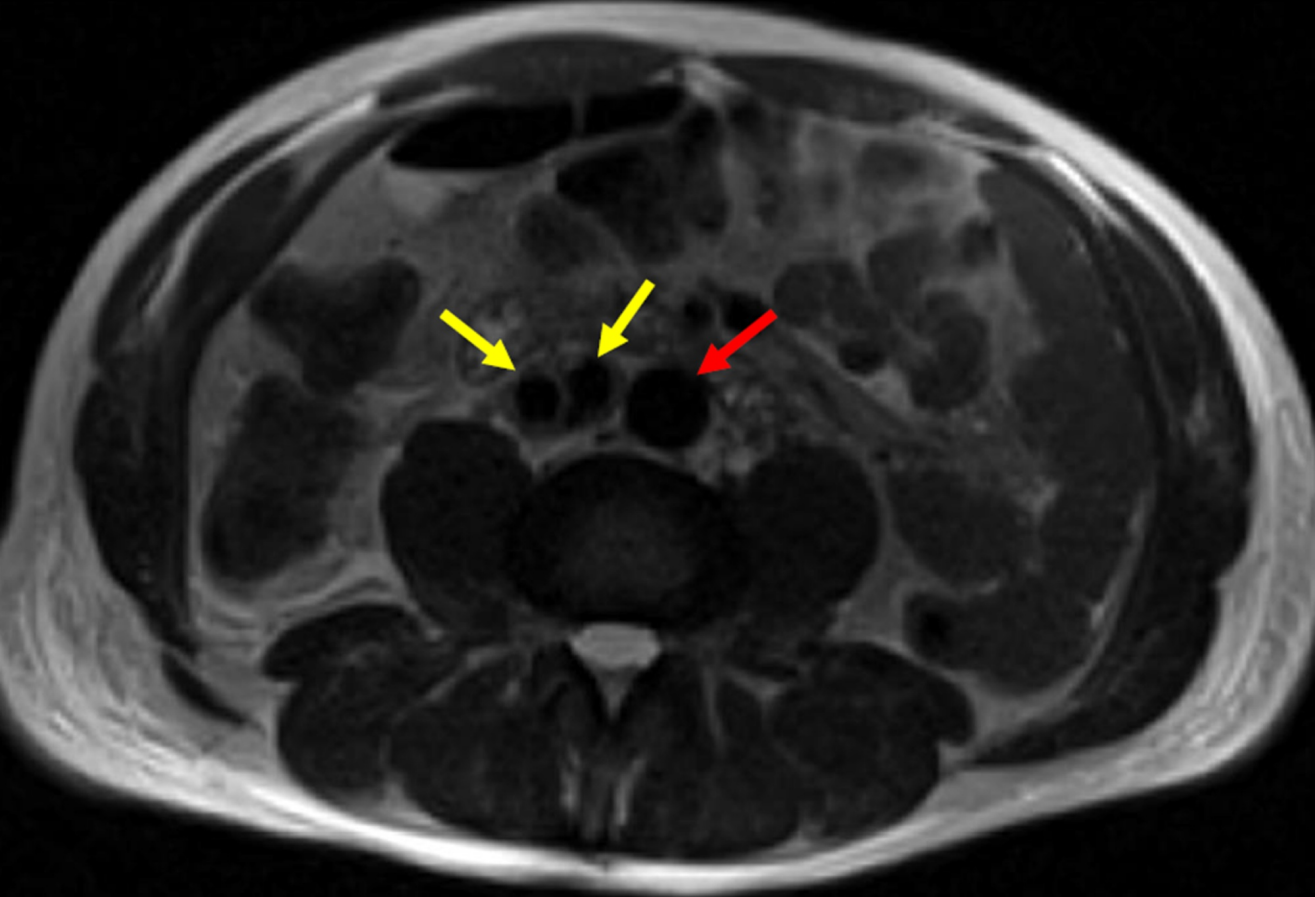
One-week Post-operative T2 Magnetic Resonance Image Displaying Stent Graft Patency within the Iliocaval Confluence A one-week post-operative axial T2 magnetic resonance image of the lower abdomen demonstrates low signal void in the bilateral iliac veins with patency through the stent graft (yellow arrows), and low signal flow void within the distal abdominal aorta (red arrow), consistent with patency.
